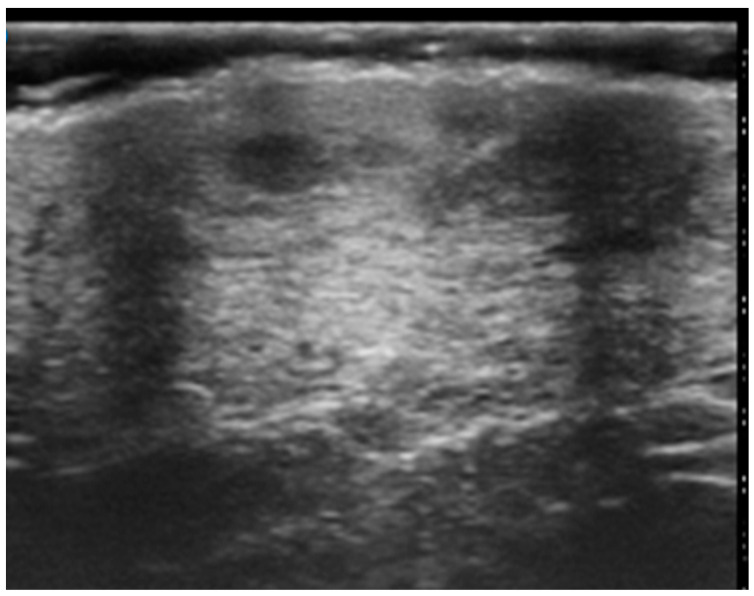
Focal pseudonodular lesion (T-cell lymphoma). Examination performed with a very high-frequency ultrasound probe at 48 MHz in the region of the right scapula: Iso-hypoechoic lesions with regular margins and epidermal localization.
